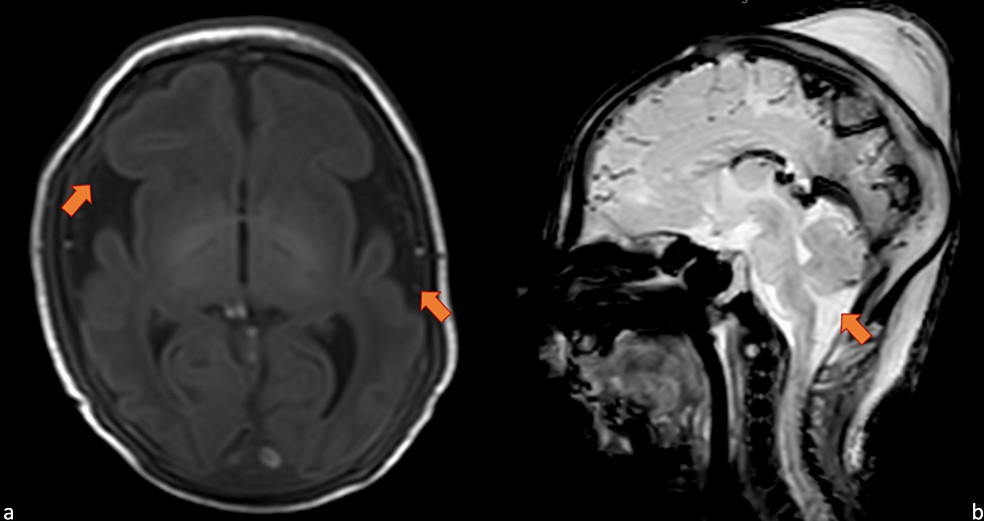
Brain MRI on day 7 of life Brain magnetic resonance imaging on day 7 of life. Frontotemporal oligogyric microcephaly and mild prominence of the pericerebral CFS space on axial T1-weighted imaging (a), large pericerebellar cisterns and cerebellar hypoplasia on sagittal T2-weighted imaging (b).
